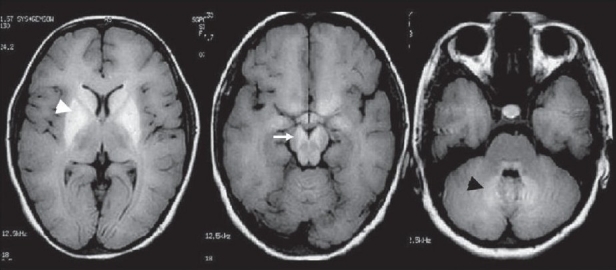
Cranial MRI on T1 sequence of the patient #3 with pseudohypoparathyroidism shows hyperintensity in the striatopallidal (white arrow head), midbrain (white arrow) and pontocerebellar region (black arrow head) whose CT scan was normal
